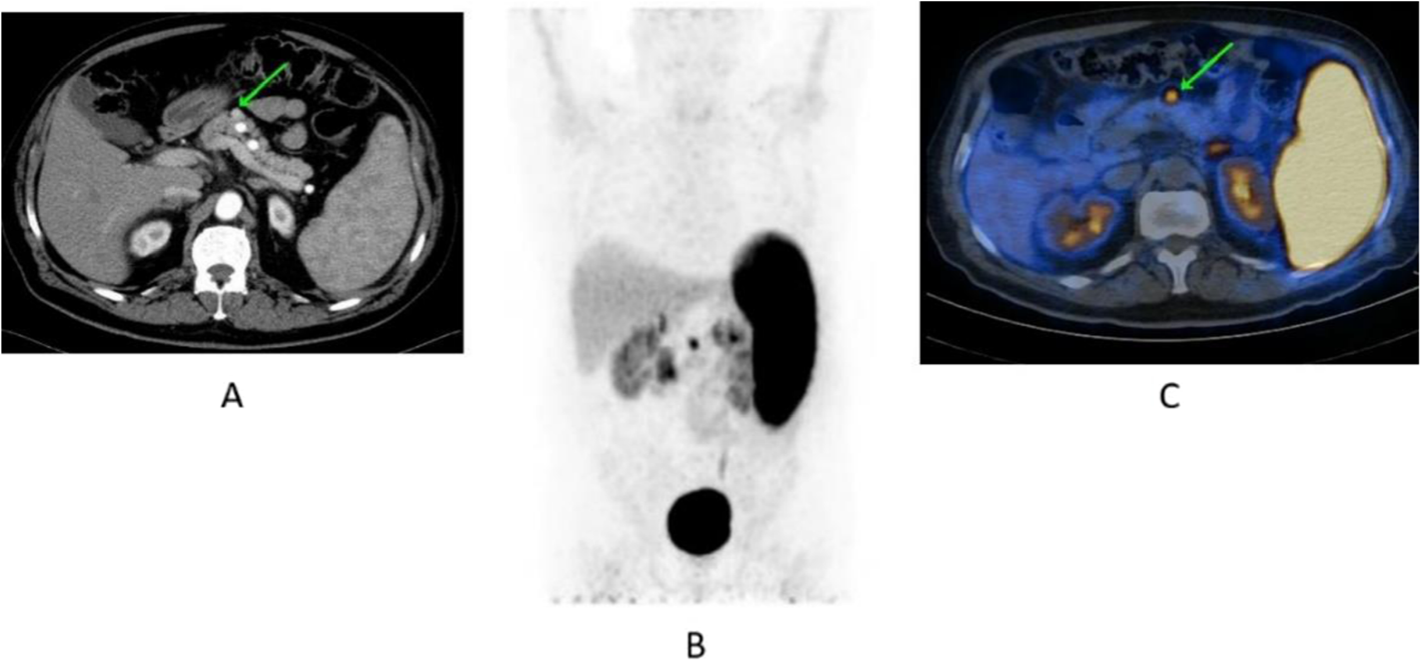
^68^Ga-DOTA peptide, suspected lesion of the pancreas, specificity. *Clinical history*: 50 y.o. woman; a hypervascular lesion in the pancreas was incidentally found on CT (a). *PET/CT findings*: focal area of high expression of somatostatin receptor analogues in the pancreas corresponding to the CT finding (b MIP, c fused). Surgery confirmed the presence of a well differentiated neuroendocrine tumour (Ki67 2%)
